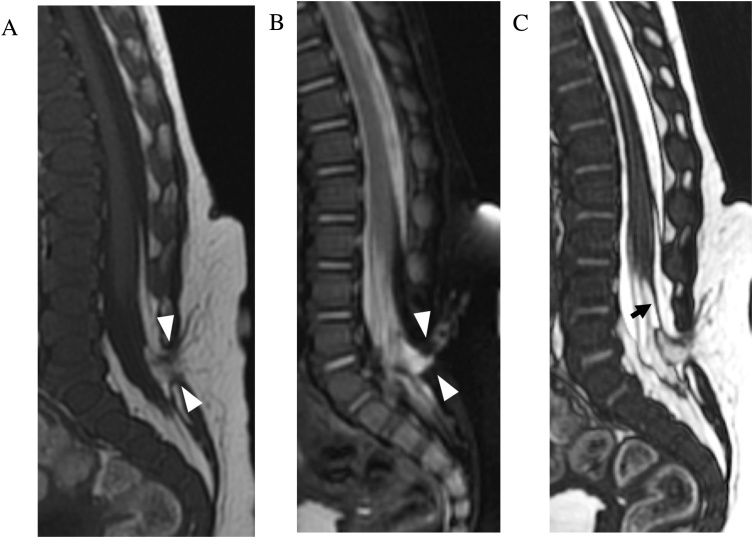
Dorsal epidural lipoma with tethered cord. 3-month-old male with palpable subcutaneous mass over the sacrum on physical exam with no cutaneous discoloration. Sagittal T1-weighted FSE (a), T2-weighted FSE (b) and FIESTA-C (c) MRI images demonstrate a spina bifida defect at L5-S1. A lumbosacral subcutaneous lipoma extends through the spina bifida defect (arrowheads) into the dorsal epidural space. Compared to T2-weighted FSE, FIESTA-C better demonstrates the filum terminale (arrow) which is tethered to the dorsal epidural lipoma and the low-lying conus.
